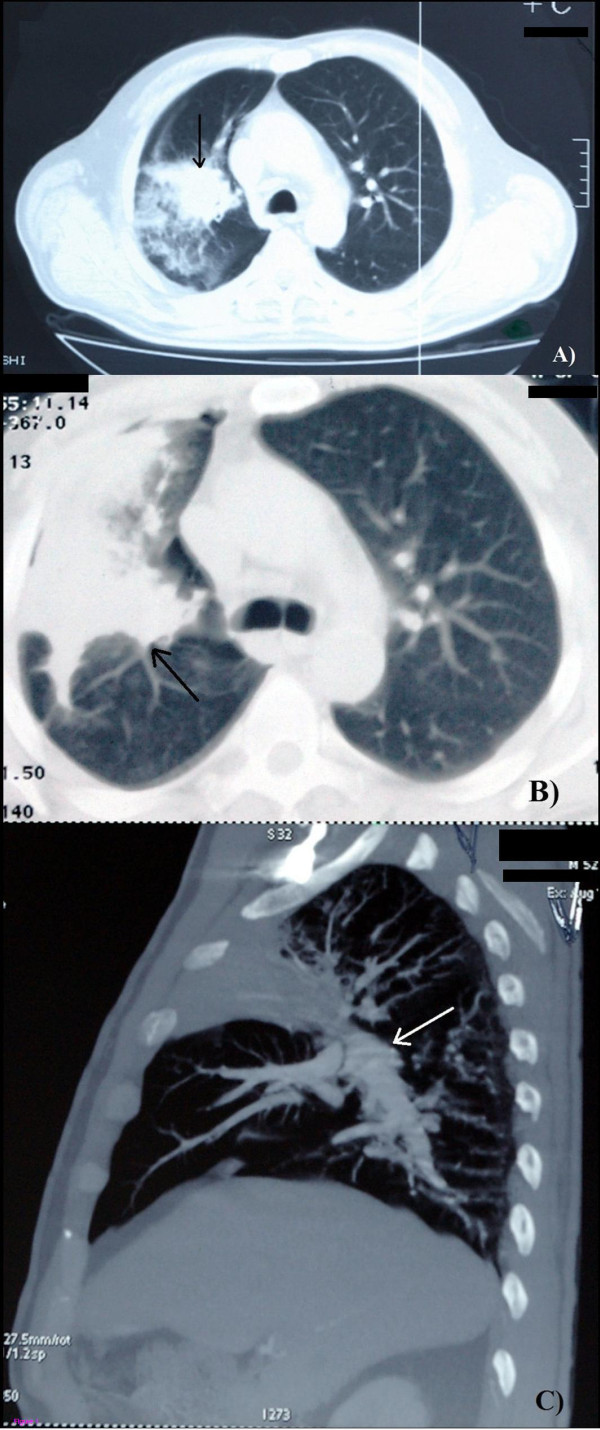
Findings of pulmonary mucormycosis detected by computed tomography (A, B) and multi-slice computed tomography (C). A) First hospitalization, soft-tissue alveolar-consolidation changes of the anterior and posterior segment of the upper lobe (arrow). B) Second hospitalization, peribronchial circular thickening, with deformation and constriction of all bronchial segments, with peribronchial propagation in the form of irregular, interlobular septal and nodular (up to 1cm) opacities (arrow). Suspected clot masses were viewed in the lumen of the superior vena cava, inferior vena cava, azygos vein and splenic vein. C) Third hospitalization, the signs of minor regression of the soft-tissue inflammatory consolidation of the upper right lobe (arrow).
